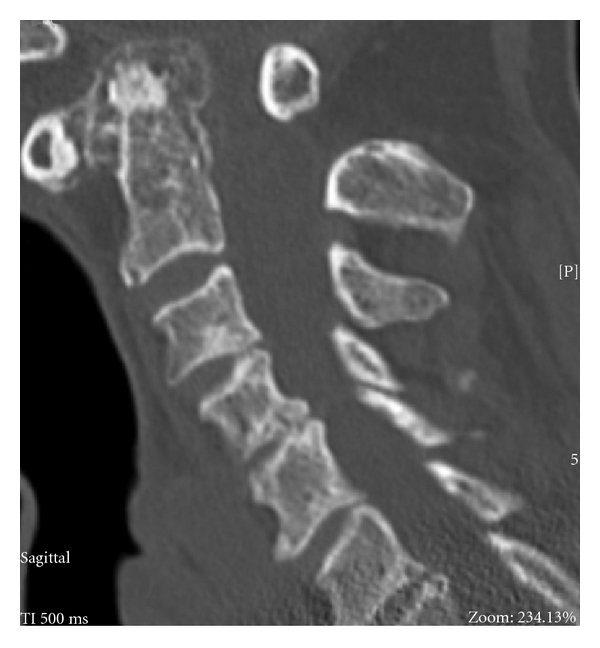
Sagittal CT scan reconstruction of a patient showing odontoid pannus reducing the diameter of the spinal canal at the level of the odontoid peg.
